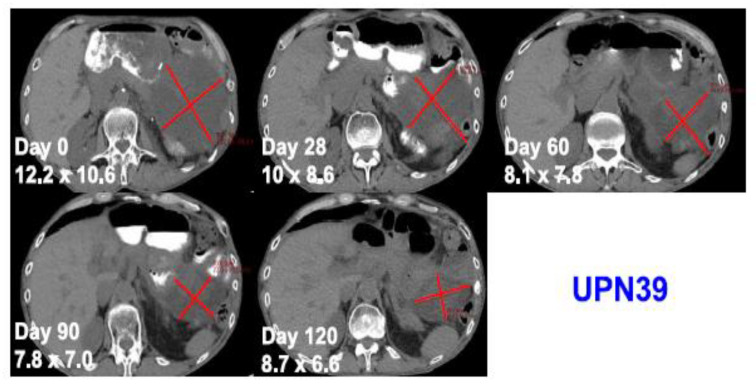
Computed tomography scans of a patient with metastatic treatment refractory liposarcoma showing tumor regression after administration of an autologous NYCE T cell. Adapted from [47] with permission from the authors.
